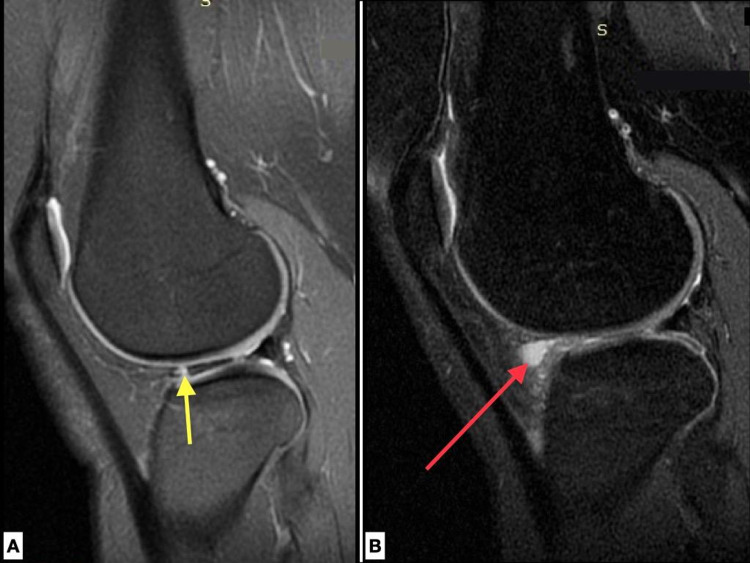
MRI pre-op and post-op. A. T2 sagittal image of the right knee of a 22-year-old female patient with a tear (yellow arrow) in the anterior horn of the lateral meniscus. B. STIR sagittal image of the same patient 12 months postoperatively, where a meniscal cyst is formed (red arrow).
